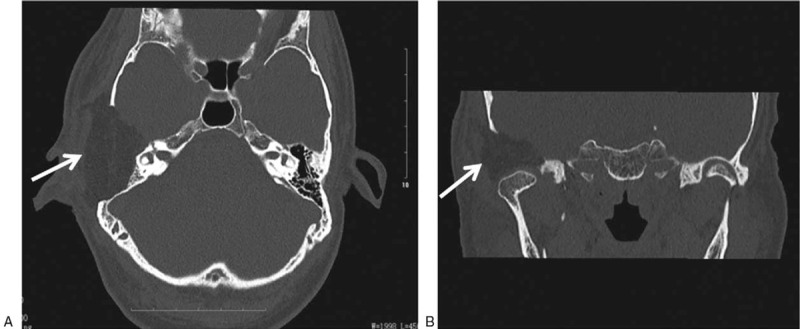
Temporal bone CT of the CMF patient after surgery. (A) The transverse section and (B) the coronal section. CMF = chondromyxoid fibroma, CT = computed tomography.
